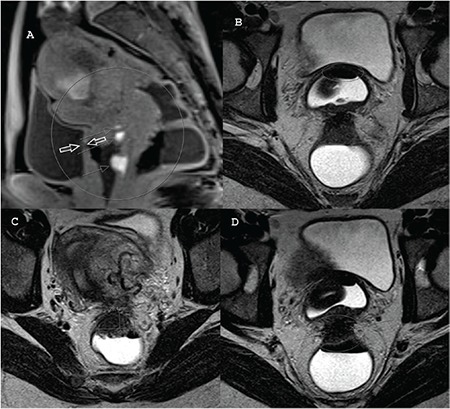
A) T1W Dixon image of patient 1 in the present magnetic resonance imaging (MRI) scan. The white arrows represent the invasion of the posterior wall of the urinary bladder by the vaginal anterior wall fibrosis. The thin arrows indicate the posterior vaginal wall lesions with subacute bleeding, B) Axial T2W image of patient 1 in the present MRI scan. The circle shows the fibrotic invasion between the urinary bladder and the vagina, C) Axial T2W image of patient 1 in the present MRI scan shows the measurement of transverse diameter of the anterior sigmoidal wall lesion, D) Axial T2W image of patient 1 in the present MRI scan. The circle and the thin arrows show the fibrotic invasion between the sigmoid bowel and the vagina
